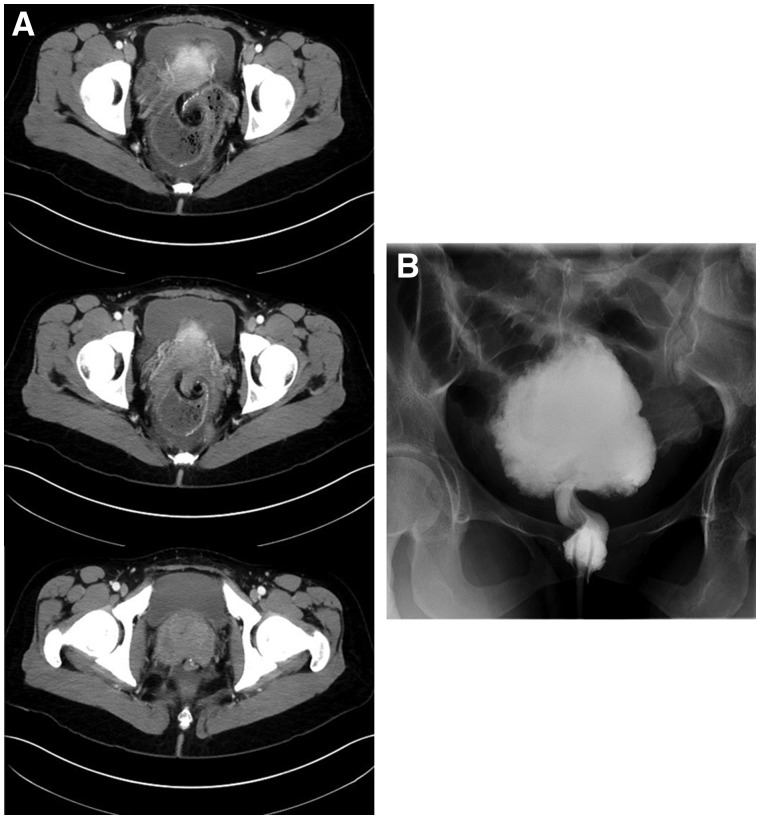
Imaging characteristics of pouch volvulus. (A) Computed tomography showing swirling of the distal pouch and its mesentery just above the ileoanal pouch anastomosis. (B) Water-soluble contrast enema showing a volvulus in the lower ileoanal pouch, with marked dilation of the proximal ileoanal pouch. On this exam, the volvulus could not be reduced with administration of Hypaque enema. The ileoanal anastomosis is patent and no leakage of contrast is observed.
